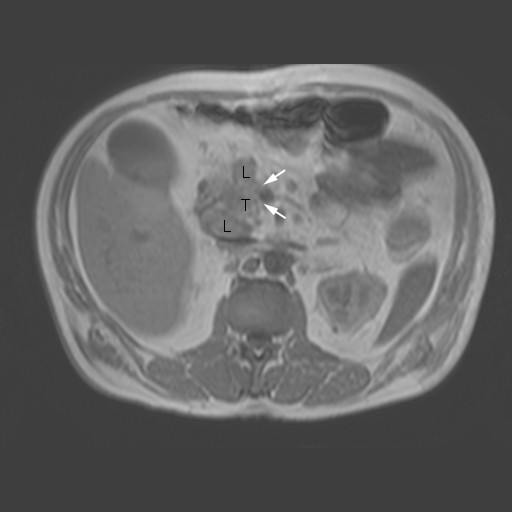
T1 weighted MRI at time of diagnosis. T = tumor; L = lymph node; arrows indicating semicircular contact to the superior mesenteric vein.
